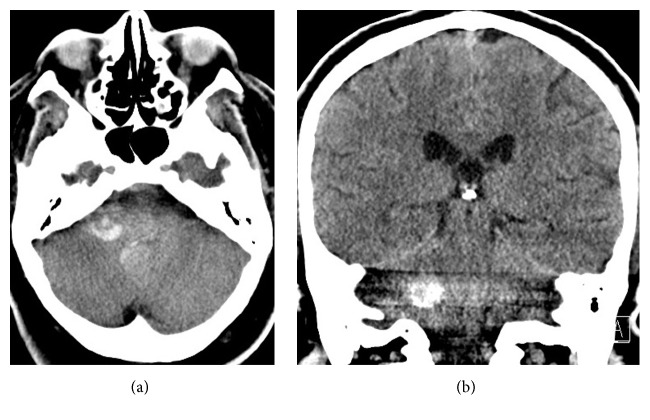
Axial (a) and coronal (b) noncontrast computed tomography (CT) of the head demonstrates hemorrhage in the region of the inferior right cerebellar peduncle, which appeared intraparenchymal, with extension into the fourth ventricle.
